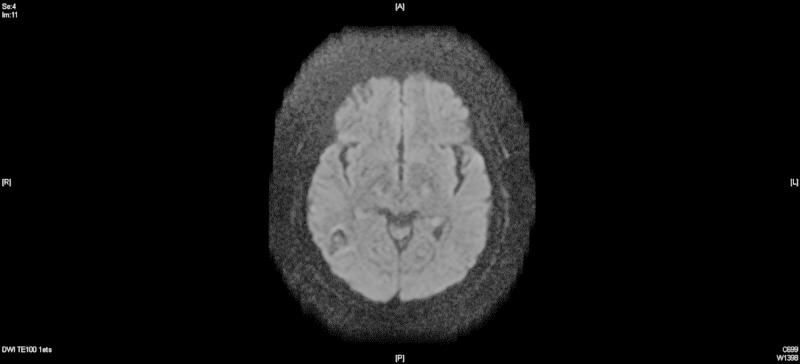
Magnetic resonance imaging (MRI) brain with contrast, day 1.
